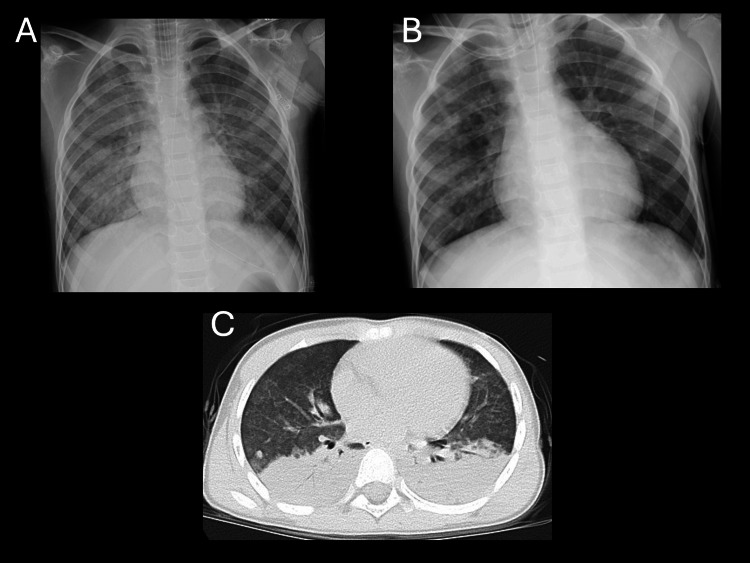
Chest radiographs and chest CT images in case 1 (A) Chest radiograph obtained before prone positioning. (B) Chest radiograph obtained after prone positioning, demonstrating improved lung radiolucency compared with panel A. (C) Chest CT image obtained before prone positioning, showing extensive dorsal atelectasis. Note: The left side of each image corresponds to the patient’s right side.
